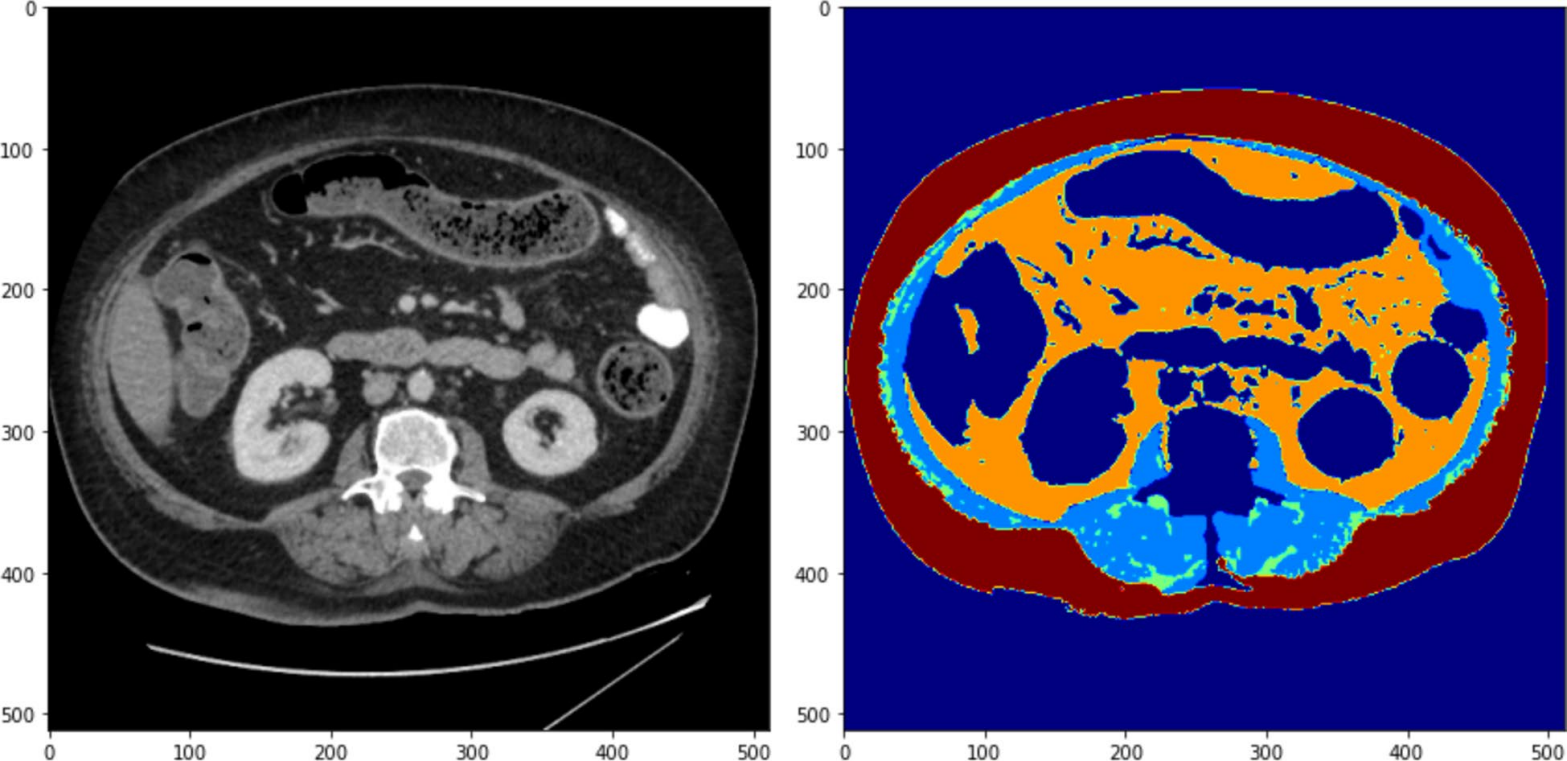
AI-generated body composition assessment of a single axial CT image. Light blue: skeletal muscle; green: intermuscular/intramuscular adipose tissue; orange: visceral adipose tissue; red: subcutaneous adipose tissue
